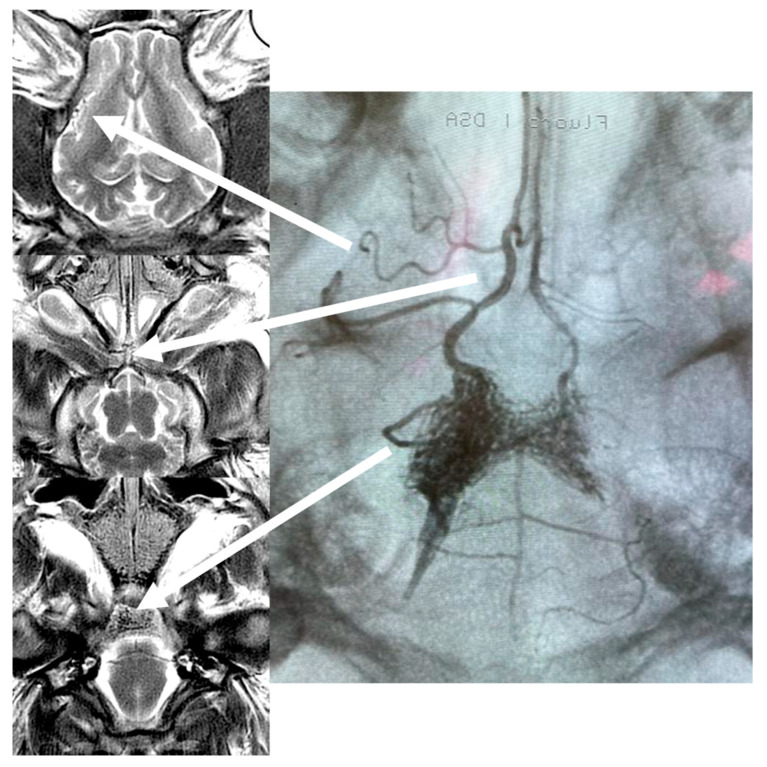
FU T2 scans 20 min after Onyx injection and an X-ray image of the Onyx cast.
